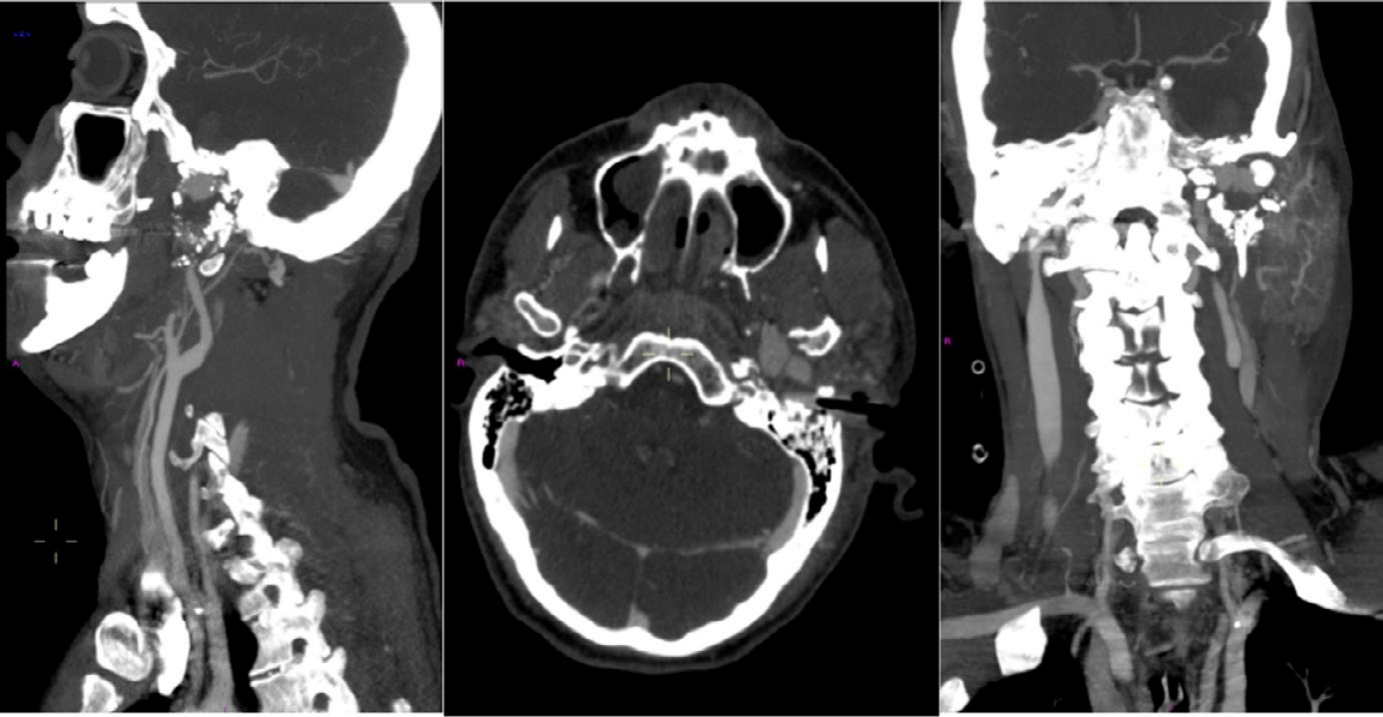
Sagittal, Axial and Coronal view of Temporal Bone CT angiography. The left ECA, left proximal middle meningeal artery, and the left pseudoaneurysm captured on key images
